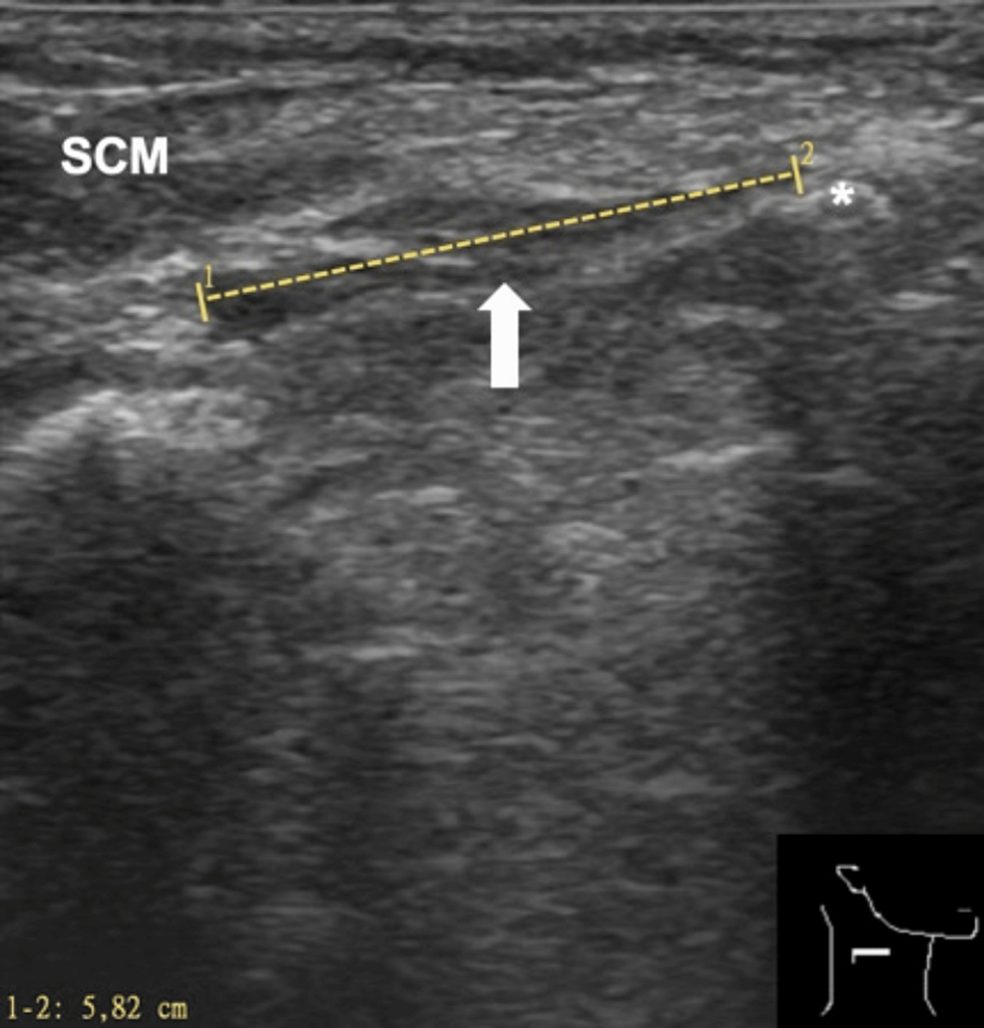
Ultrasound image of the right neck displaying the extent of the entire accessory muscle. Arrow, accessory muscle; SCM, sternocleidomastoid muscle; asterisk, corpus of the hyoid bone
